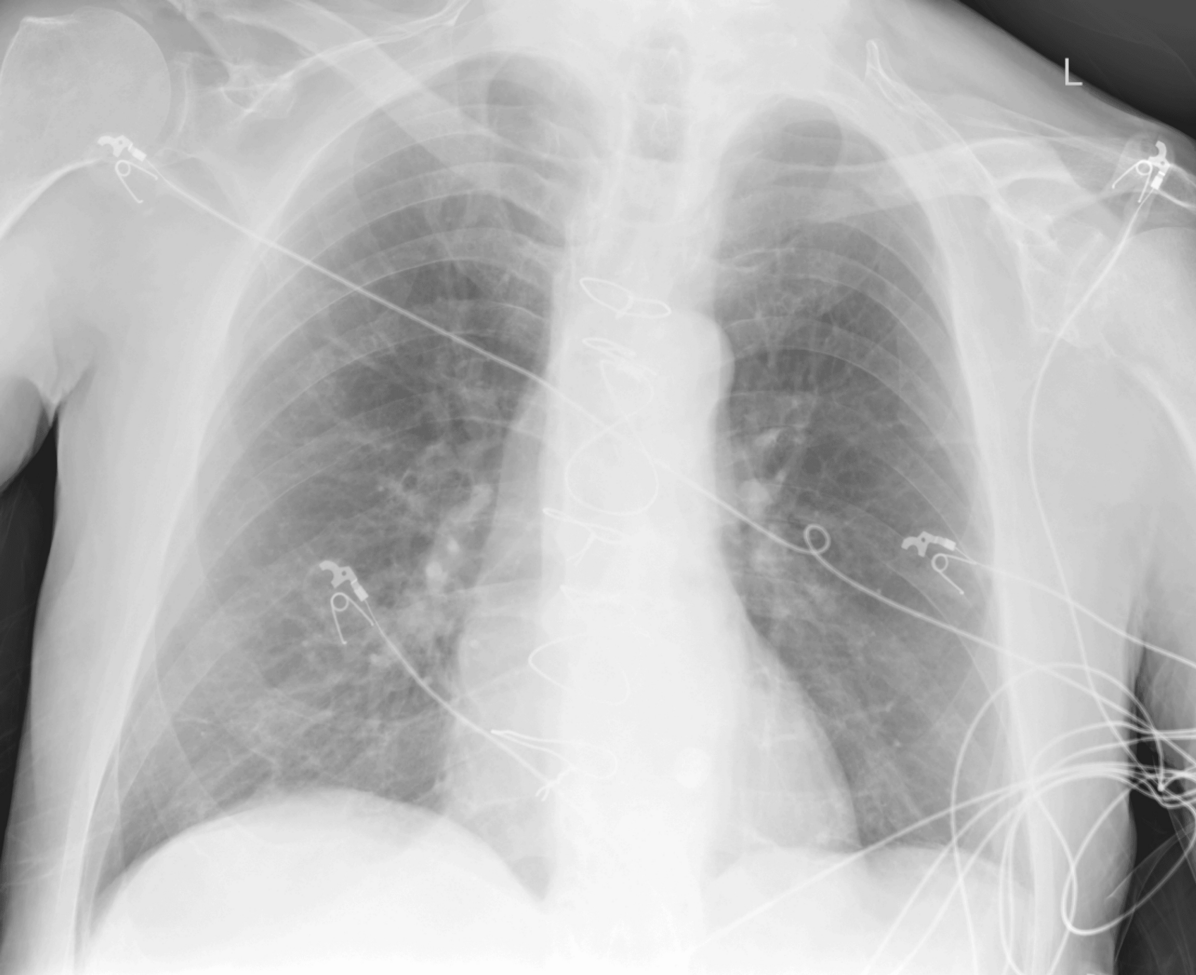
Portable chest X-ray showing no acute abnormalities
